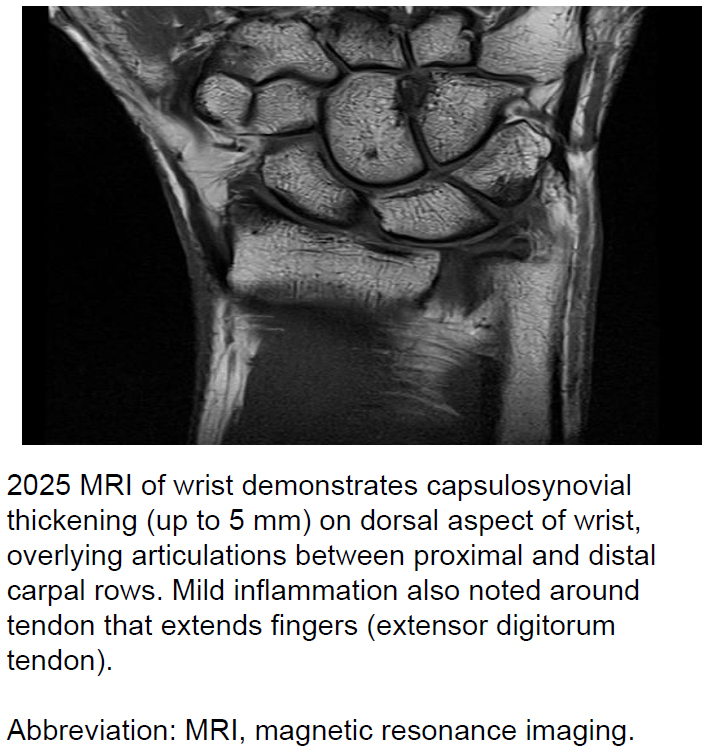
2025 MRI Image of Ross River Virus-Infected Australian Service Member's Right Wrist Indicating Capsulosynovitis
